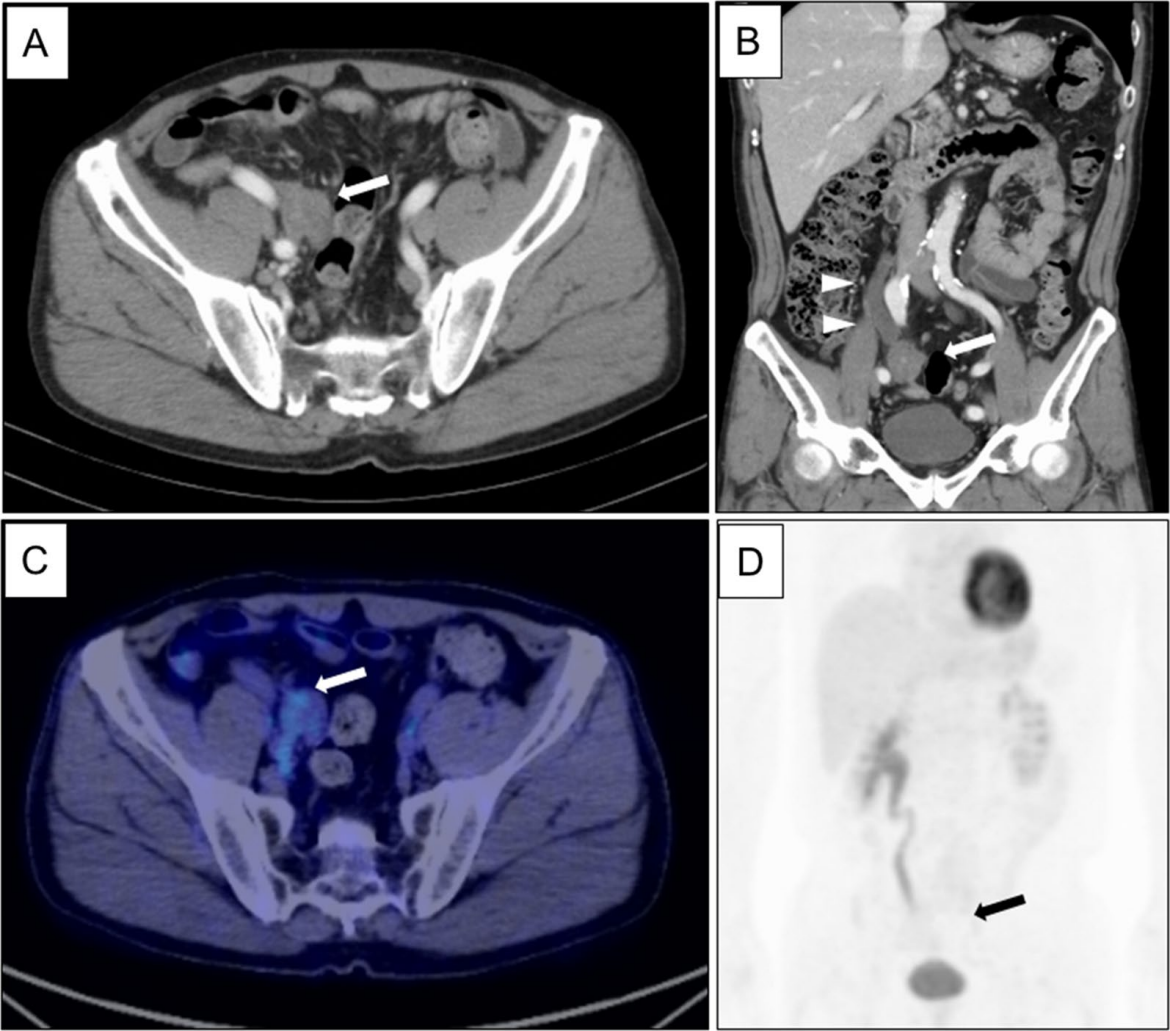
Contrast-enhanced computed tomography (CT) and positron emission tomography/computed tomography (PET/CT). Contrast-enhanced computed tomography (CT) scan showed a mass-forming lesion on the right external iliac artery (43 × 26 mm) (A arrow, B arrow) and hydronephrosis (B arrowhead). Positron emission tomography/computed tomography (PET/CT) showed a mass-forming lesion without high accumulation (C arrow), which obstructed the ureter (D arrow)
